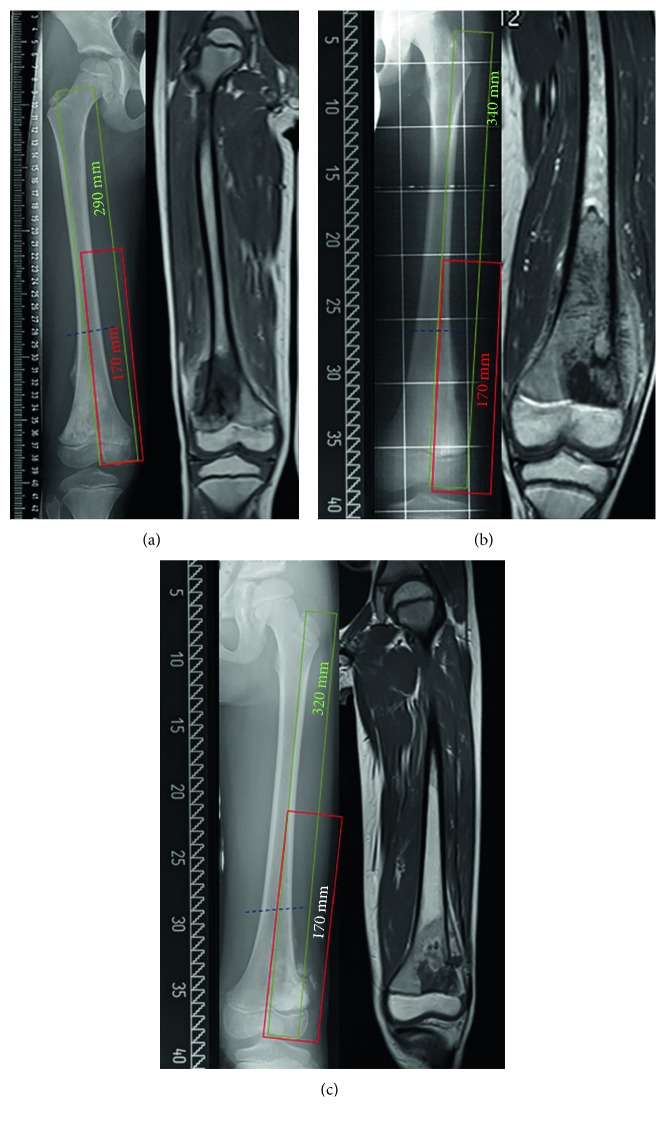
X-ray and MRI showing three case scenarios including specific answers for treatment options. None of the cases involved skip metastasis, metastatic disease, intra-articular tumour infiltration, or pathological fracture. (a) Male, 6.5 years at initial diagnosis, osteosarcoma of distal femur, and femur length (greater trochanter to eminentia intercondylaris) is 290 mm. (b) Female, 8.0 years at initial diagnosis, osteosarcoma of distal femur, and femur length (greater trochanter to eminentia intercondylaris) is 340 mm. (c) Male, 10.5 years at initial diagnosis, osteosarcoma of distal femur, and femur length (greater trochanter to eminentia intercondylaris) is 320 mm.
